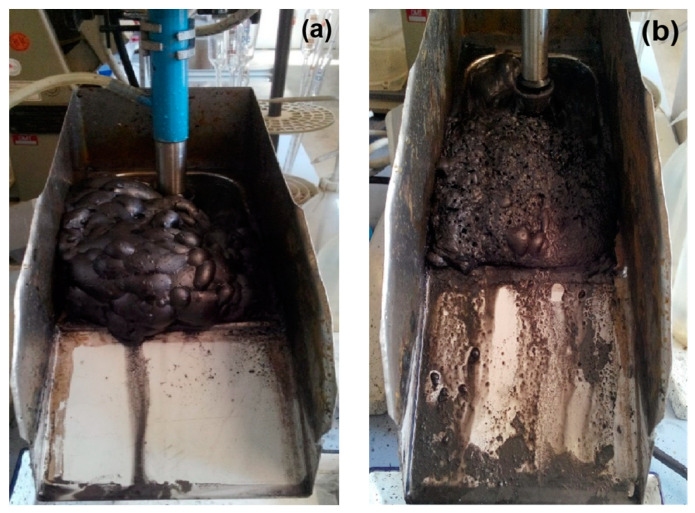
Photos of the coal froths: (a) conventional; (b) ultrasonic [140].
